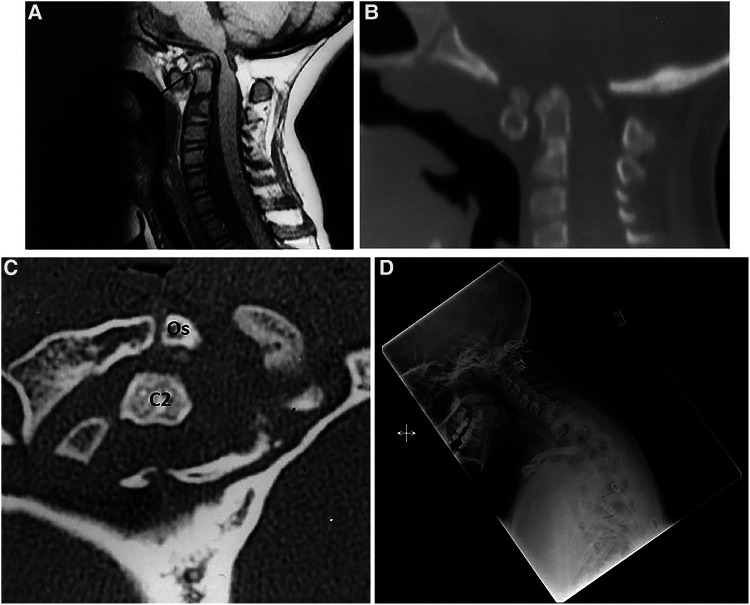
(A) Midsagittal T1-W MRI of CVJ in a 6-year-old child. Note the cervicomedullary compression by the superior C2 and the dorsal C1 arch. There is an os odontoideum. The arrow demonstrates the cruciate ligament. (B) 2D CT of CVJ of the patient in (A). (C) Axial CT at C1. The anterior arch of C1 is bifid with the os odontoideum in the bipartite space. (D) Lateral cervical radiographs obtained 11 years later. Note the normal alignment and dorsal occipitocervical fusion.
